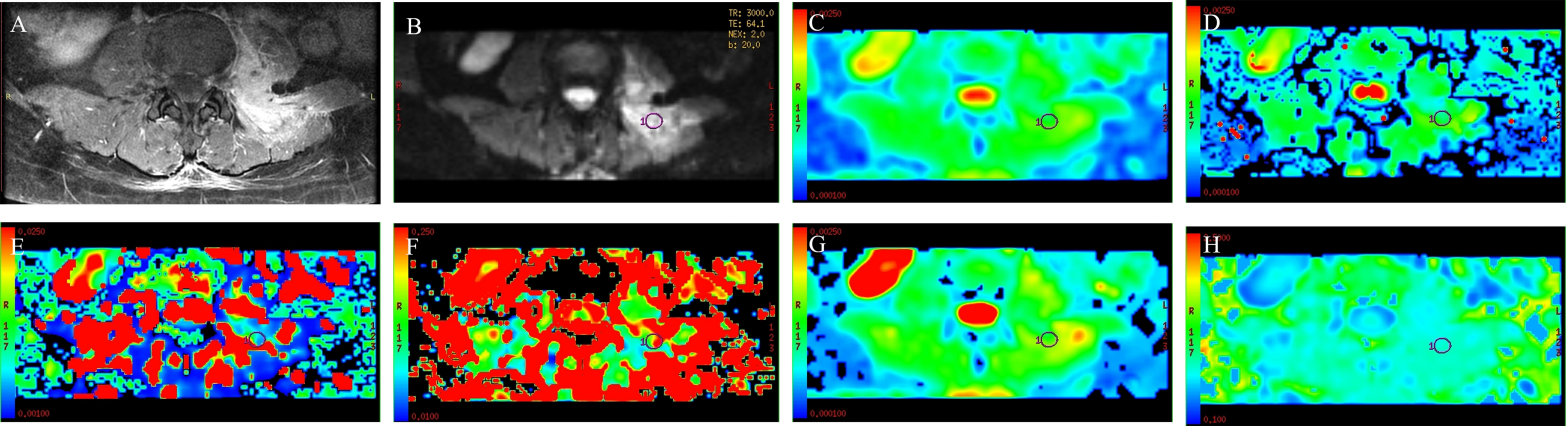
A 53-year-old man with spinal metastasis from lung cancer. The lesion showed obvious enhancement on T1WI (A) and hyperintensity on DWI (B). ADC values were 0.926 × 10^−3^ mm^2^/s (C). The ROI was placed on DWI (B) and copied to the other IVIM parametric maps of ADC_slow_, ADC_fast_, f, DDC, and α (D–H) with values of 0.8 × 10^−3^ mm^2^/s, 0.0211 mm^2^/s, 0.139, 0.945 × 10^−3^ mm^2^/s, and 0.759, respectively.
